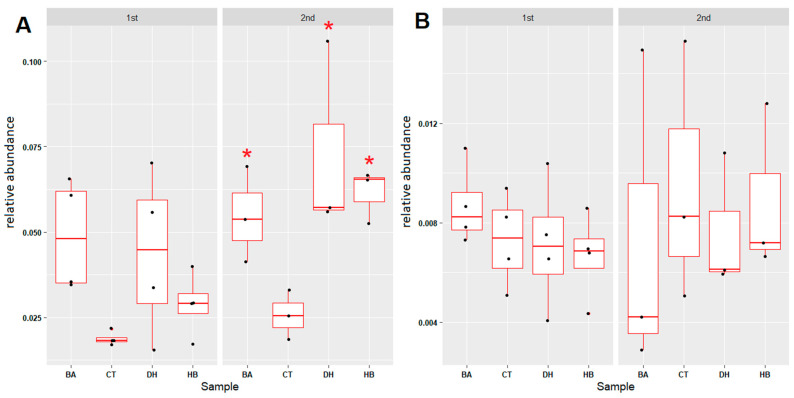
Overall relative abundance changes in microbial communities in the BA-, HB- and DHA-treated river sediments. (A) Microbial genera associated with xenobiotic degradation. (B) Microbial genera with potential pathogenic bacteria. The term “1st” indicates the period between the 0th week to the 8th week; “2nd” indicates the period between the 8th week to the 17th week. Red stars indicate the *p*-value of the Mann–Whitney U test < 0.05 (compared with control (CT)). CT: control; BA: benzoic acid; HB: 4-hydroxybenzoate; DH: dehydroacetic acid.
